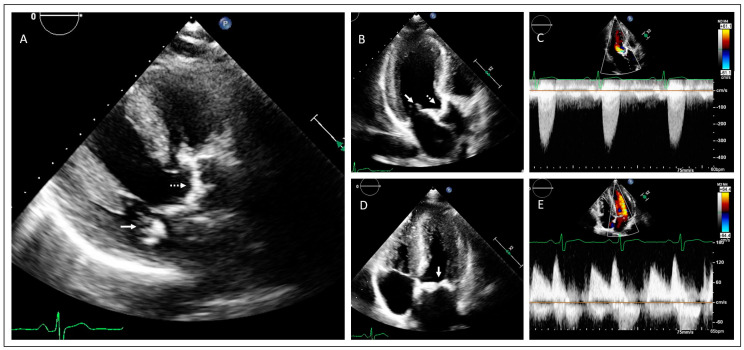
Transthoracic echocardiographic examination of family member II.2 of family 2 showing aortic valve stenosis and mitral valve calcification. (A) Parasternal long axis view and (B) apical 3-chamber view with (C) continuous wave Doppler over the aortic valve, demonstrating the aortic calcification and stenosis (dotted arrow). (D) Apical 4-chamber view with (E) continuous wave Doppler over the mitral valve, showing mitral valve calcification and stenosis (continuous arrow).
